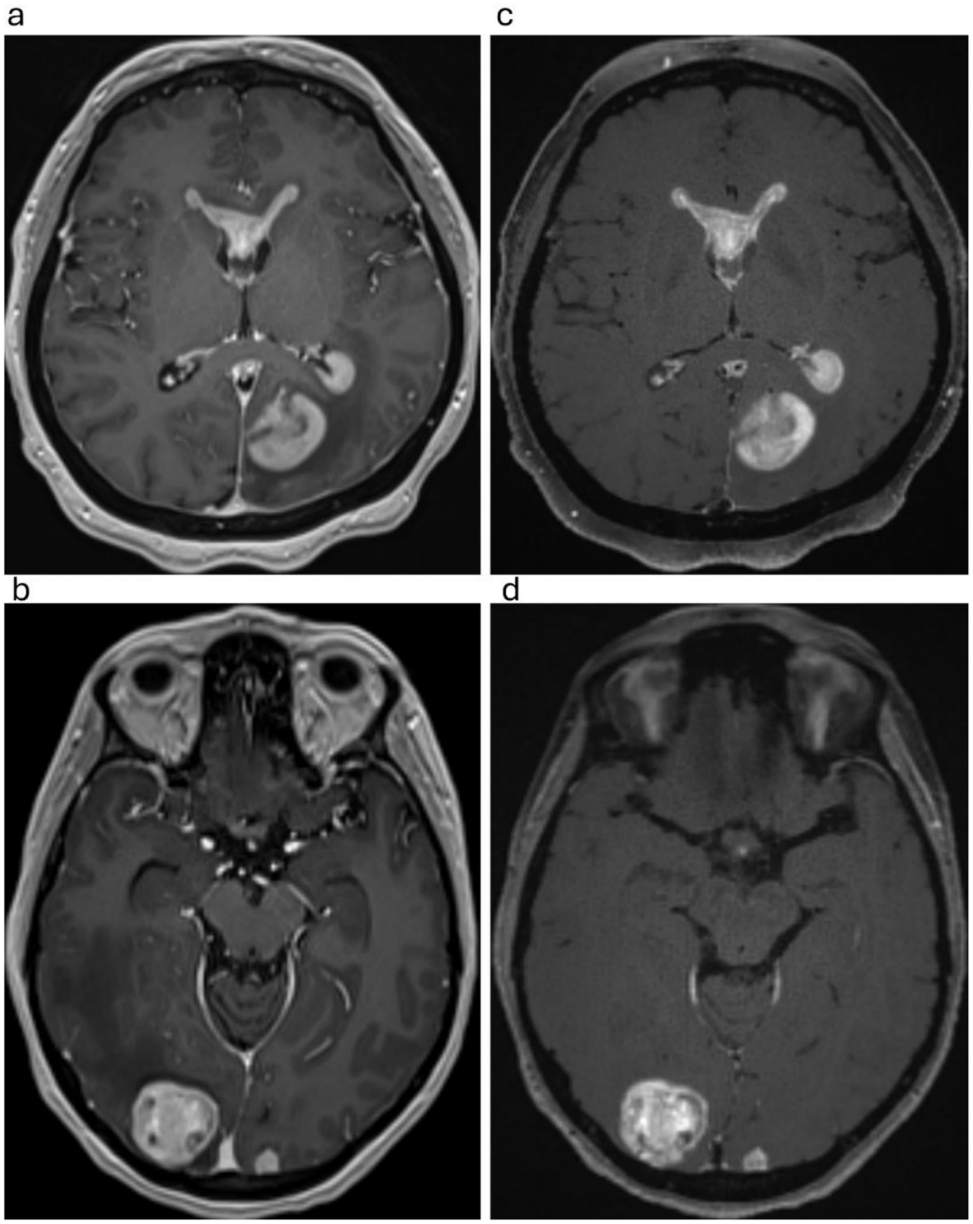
46-year-old patient with B-cell lymphoma (a, c) and 43-year-old patient with metastatic sarcoma (b, d). The comparison of axial plane images highlights the key differences between the two MRI pulse sequences: MPRAGE (a, b) and SOS echo-uT1RESS (c, d). MPRAGE provides excellent grey-white matter differentiation and clear delineation of peri-lesional changes, while the “background” brain tissue in SOS echo-uT1RESS appears more uniform. Additionally, SOS echo-uT1RESS provides dark blood features
